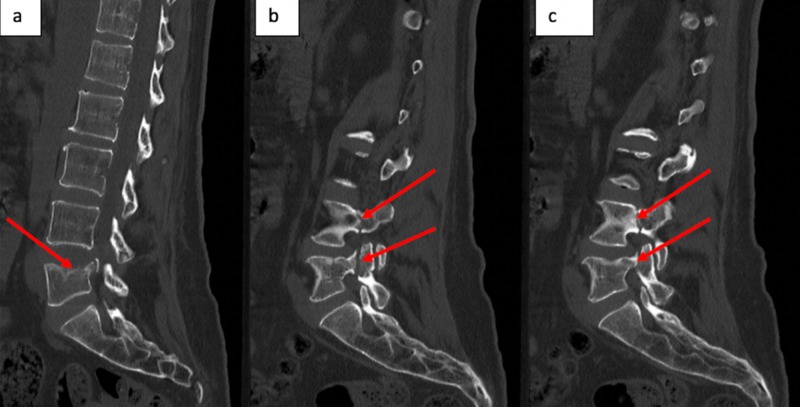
CT of the spine Non-contrast CT lumbar spine of a midline sagittal cut, which demonstrates the lumbar 5 compression fracture with retropulsed superior posterior fragment (a). Lateral sagittal images demonstrating left and right pedicle fractures at lumbar 4 and lumbar 5 (b and c).
